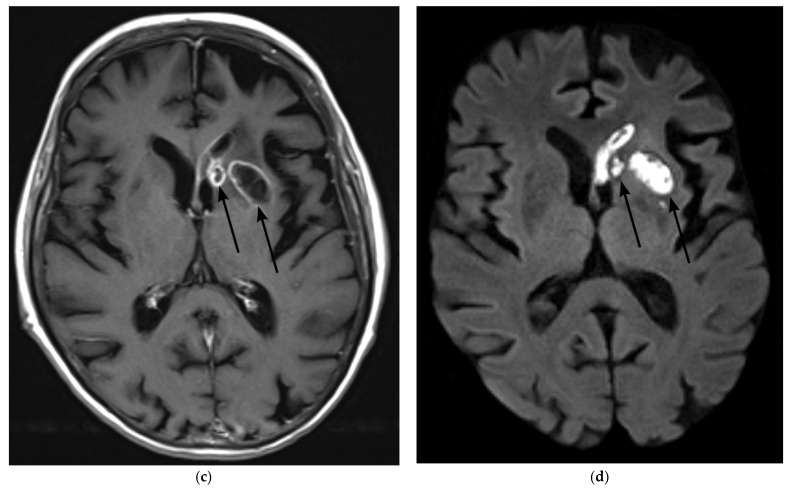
Magnetic Resonance Imaging (MRI) on day 37 after initial admission to the hospital (a) Fluid attenuated inversion recovery (FLAIR) shows lesions with mixed T2 signal (central hypointense areas with perifocal hyperintense rim) (arrow) and with surrounding edema indicated by laminar T2-hyperintense signal (star) at the anterior horn of the left lateral ventricle. (b,c) Contrast-enhanced T1-weighted image in coronal (b) and axial (c) angulation show multiple centrally hypointense ovoid lesions with rim-enhancement (arrows) (d) b-1000 image of diffusion-weighted imaging shows restricted diffusion within the lesions (arrows), indicating an abscess.
